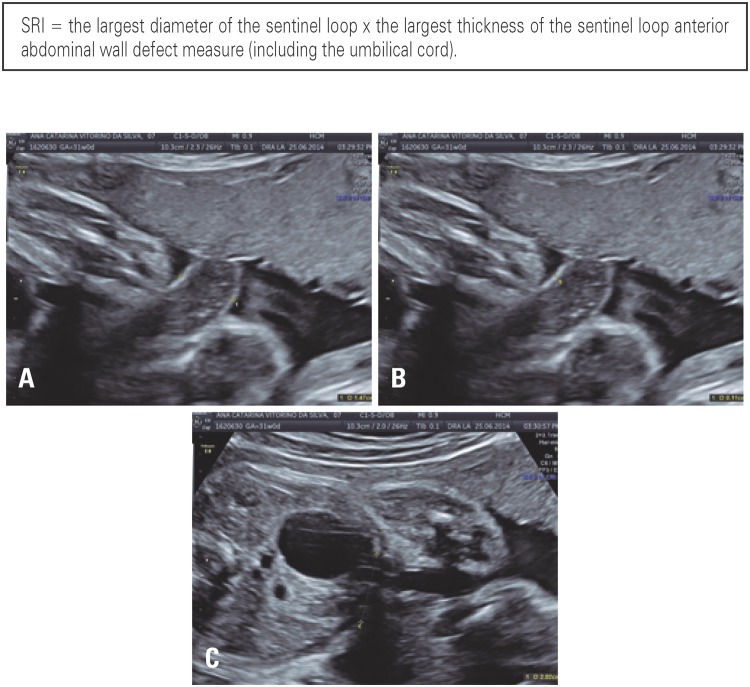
Ultrasonographic images to calculate the Svetliza Reducibility Index. (A) The largest diameter of the sentinel loop; (B) the largest thickness of the sentinel loop; (C) anterior abdominal wall defect measure (including the umbilical cord)
